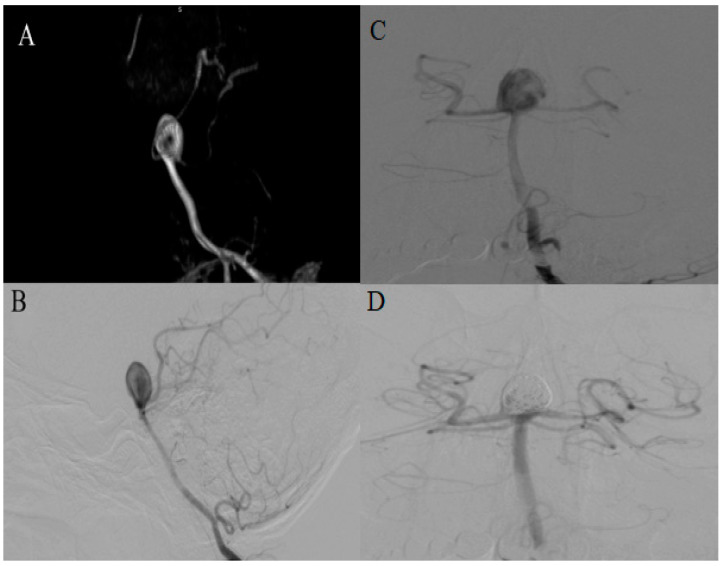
(A) MRI-TOF-3D reconstruction; (B) Preprocedural DSA of the posterior circulation in the lateral projection highlighting the morphological transformation of the initially donut-shaped aneurysm after DAPT preparation due to partial dissolution of central thrombus; (C) preprocedural DSA in the AP projection; (D) Postprocedural DSA after implantation of the pCONus2 device and coiling.
